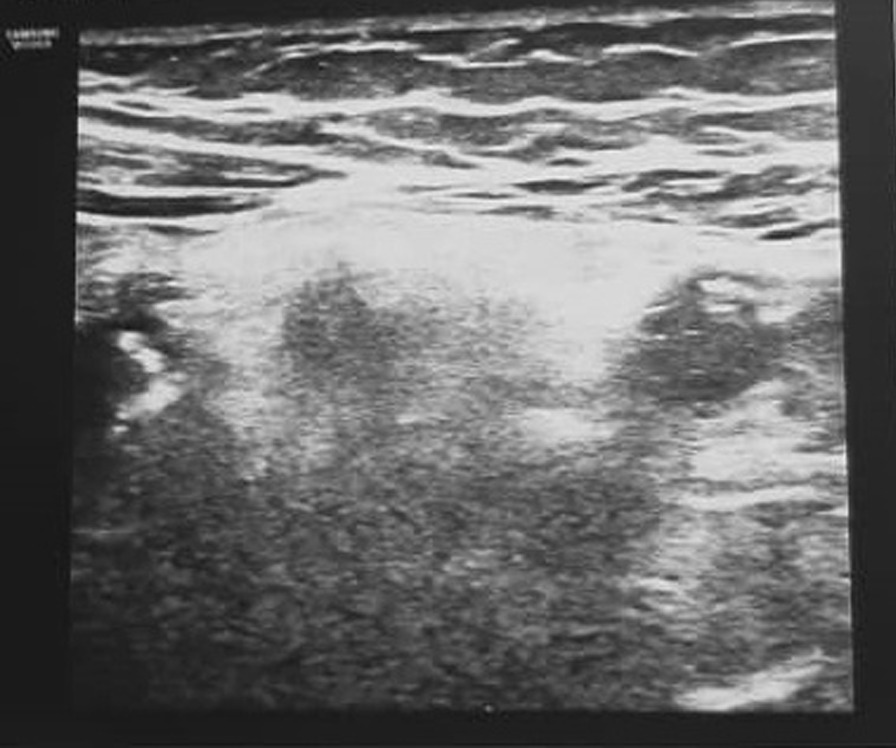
Ultrasonography showing hyperechogenicity of pericecal fat with mild free fluid in the interloop at the site of tenderness in the right lower quadrant
